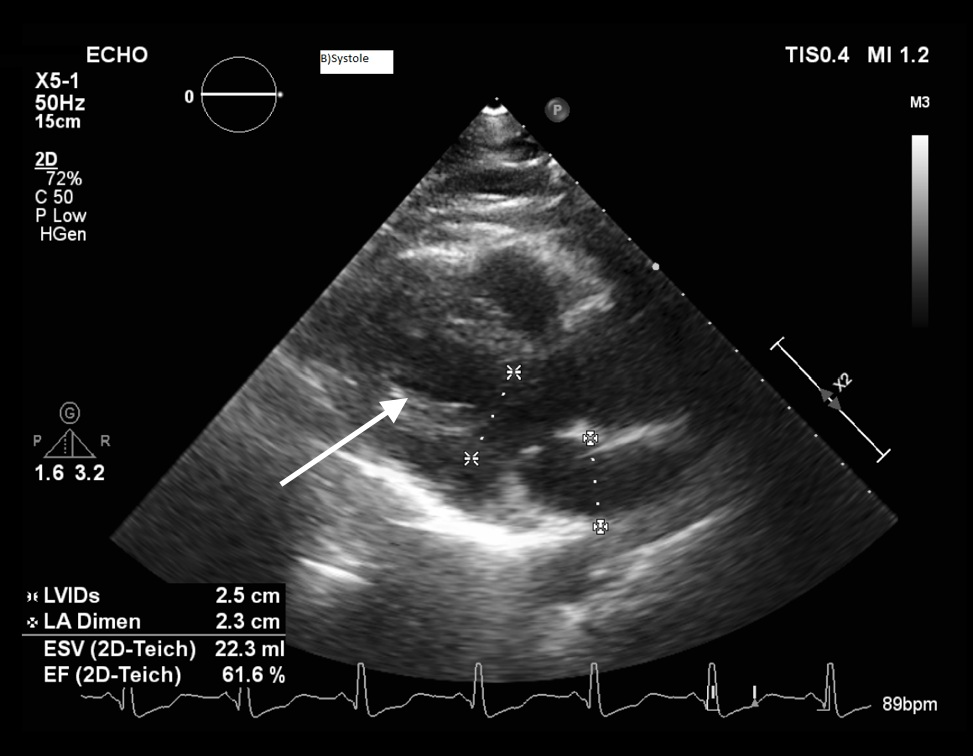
TTE obtained on day 2 of admission showing an improved ejection fraction of 55%. The arrow points to improved left ventricular contraction. TTE - Transthoracic echocardiogram
